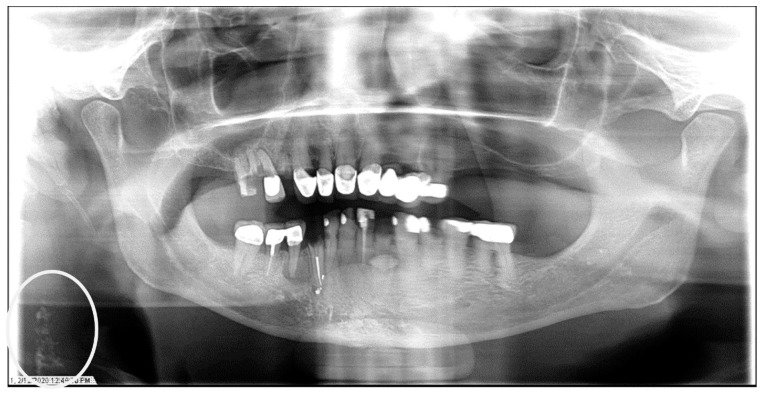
The panoramic radiograph of a 67-year-old male patient with a medical history of hypertension, diabetes mellitus, and coronary artery disease showing a unilateral right carotid artery calcification (CAC) during a comprehensive dental examination. The CAC is encircled by a white line.
